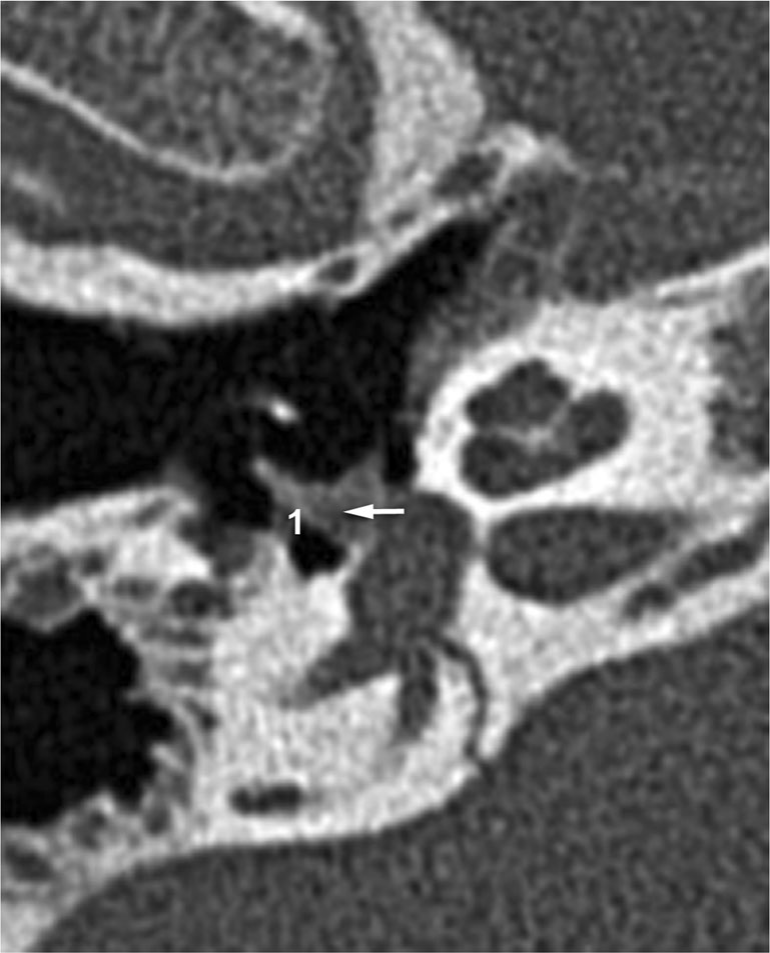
Stapedio-vestibular luxation: CT stapes view highlighting stapedio-vestibular luxation. The perilymphatic liquid leaking through the oval window (1) implies rupture of the annular ligament
